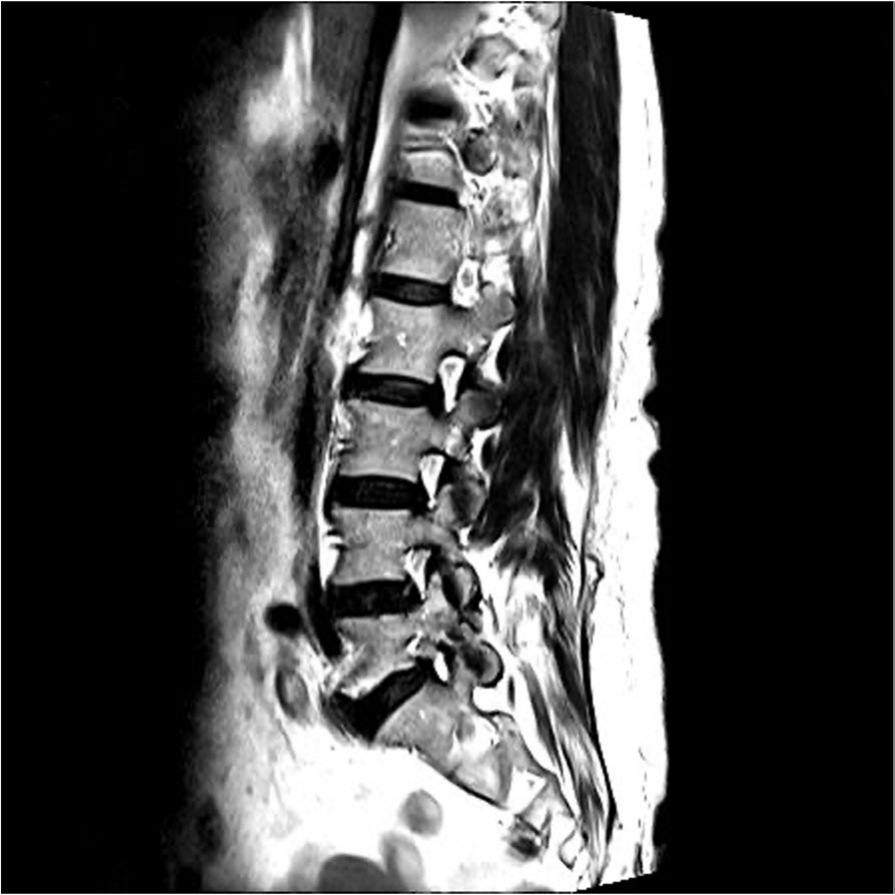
The sagittal view of MRI were effective in revealing the foraminal stenosis
